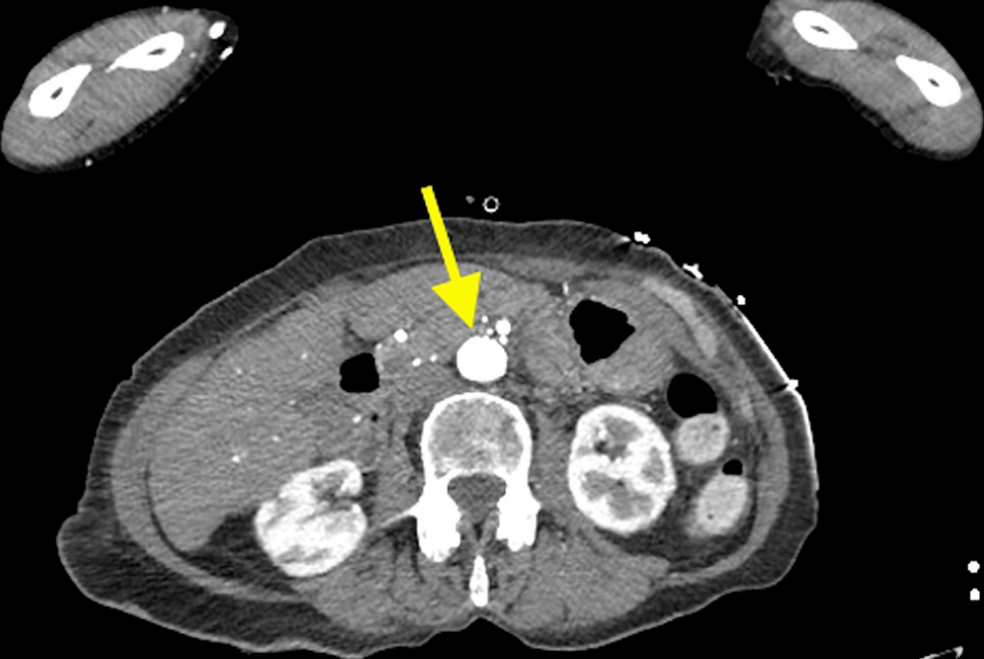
An axial, contrast-enhanced CT image of the abdominal aorta revealed no evidence of aortic dissection and aneurysm. Note a normal calibre aorta with no wall thickening or peri-aortic inflammatory stranding (arrows).
